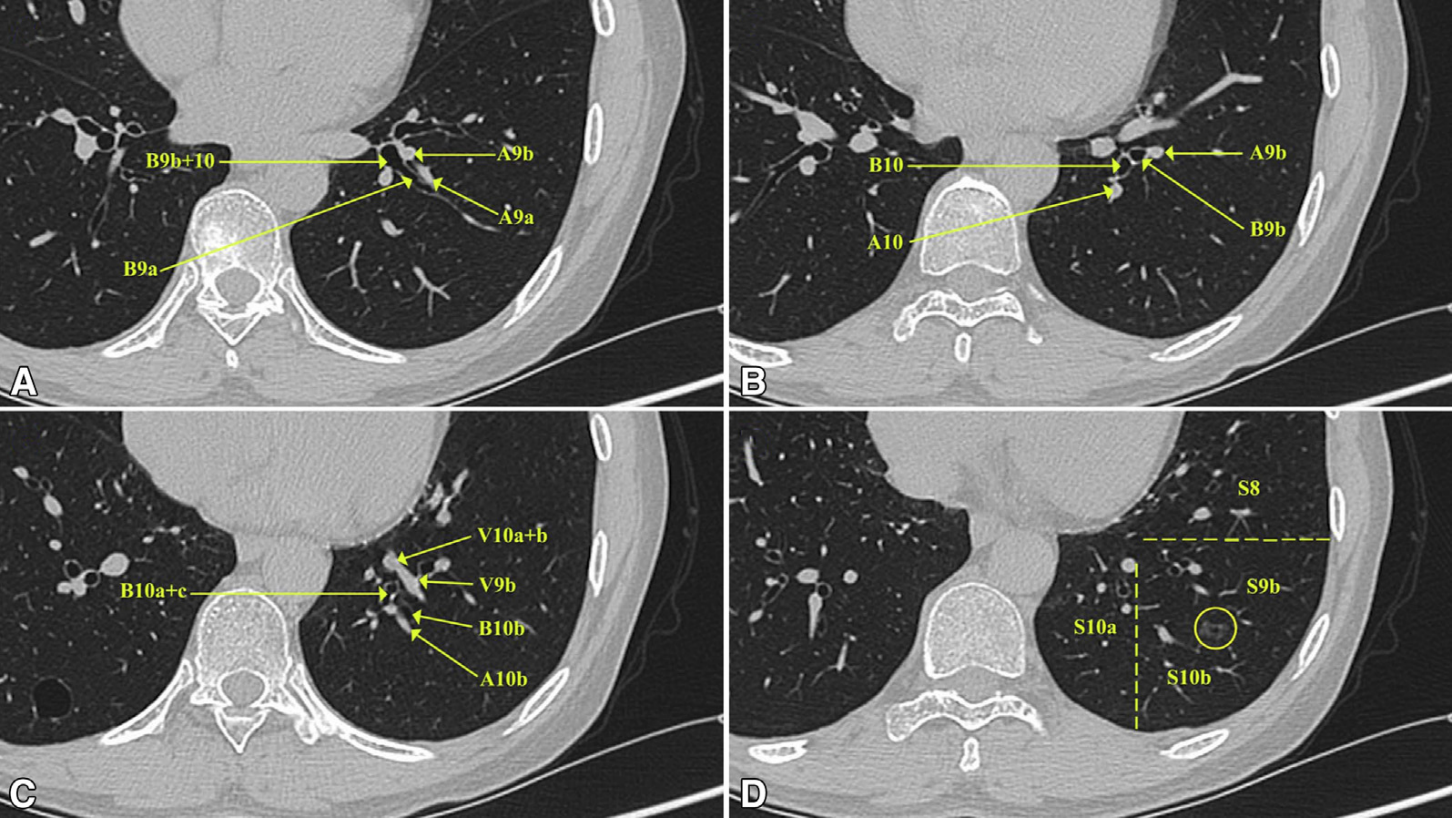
Identifying the subsegmental structures of the left basal segment and their positional relations on preoperative HRCT. A, Identifying B9a and A9a. B, Identifying B9b and A9b. C, Identifying B10b, A10b, and the intersubsegmental vein (V9b). D, Showing the lesion located near the intersubsegmental border between S9b and S10b.
